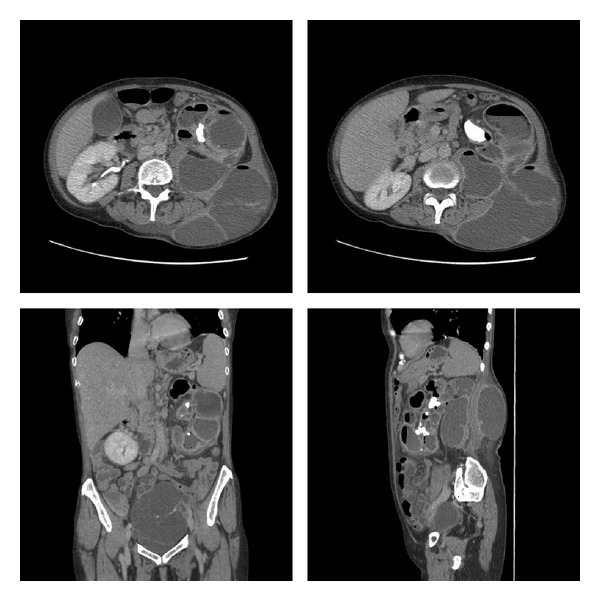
CT abdomen/pelvis with IV contrast and delayed phase demonstrating a left kidney with parenchyma replaced with multiple large hypodense collections containing fluid and gas, a left staghorn calculus, and a communication between the kidney and large flank collection. Additionally, a 13.5 × 7.7 cm multiloculated pelvic mass was noted.
